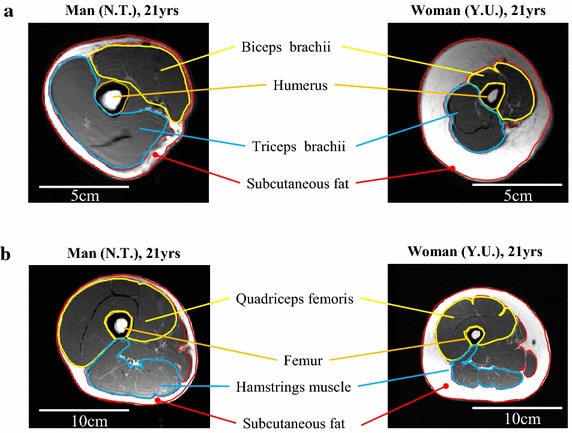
Representative axial magnetic resonance images of the *upper arm* (a) and *thigh* (b). *Left column* showing the man and *right column* showing the woman. The location of the image of the *upper limb* is at the level of the largest anatomical cross sectional area (ACSA) of the *upper arm* muscle. That of the *lower limb* is the ACSA at the level of 50% of the *thigh*. Subcutaneous fat, triceps brachii muscle, biceps brachii muscle, quadriceps femoris muscle, hamstrings muscle, humerus, and femur are outlined.
